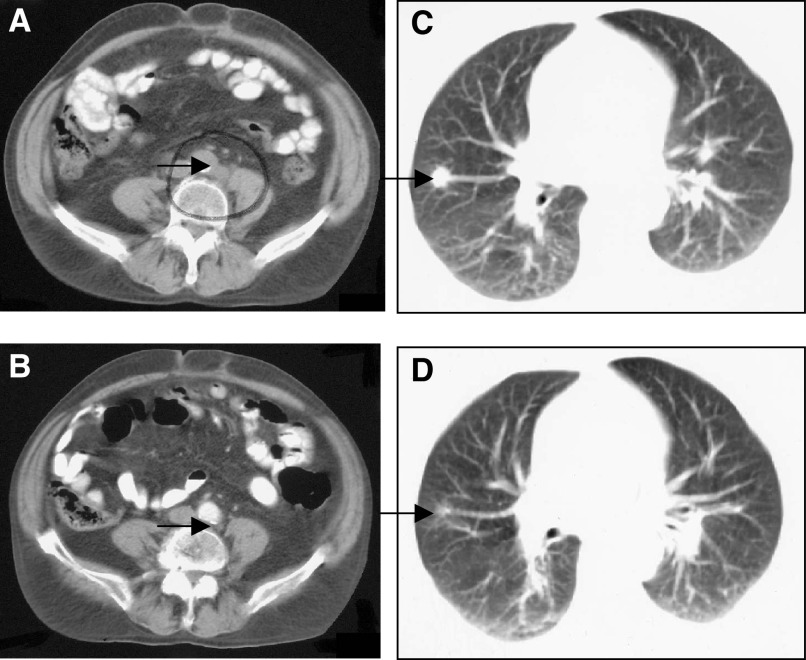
Radiological response assessment. (A) Pretreatment-enhanced axial CT scan through mid-abdomen demonstrates enlarged para-aortic nodes between aorta and left psoas muscle (arrow). Post-treatment scan at same level (B) demonstrates regression of nodal disease, a tiny focus of residual density is apparent (arrow). (C) Pretreatment axial CT through thorax demonstrates a measurable soft tissue nodule in the right middle lobe on lung window settings (arrow); post-treatment (D) the nodule has reduced in size, a small ill-defined density is still apparent (arrow).
